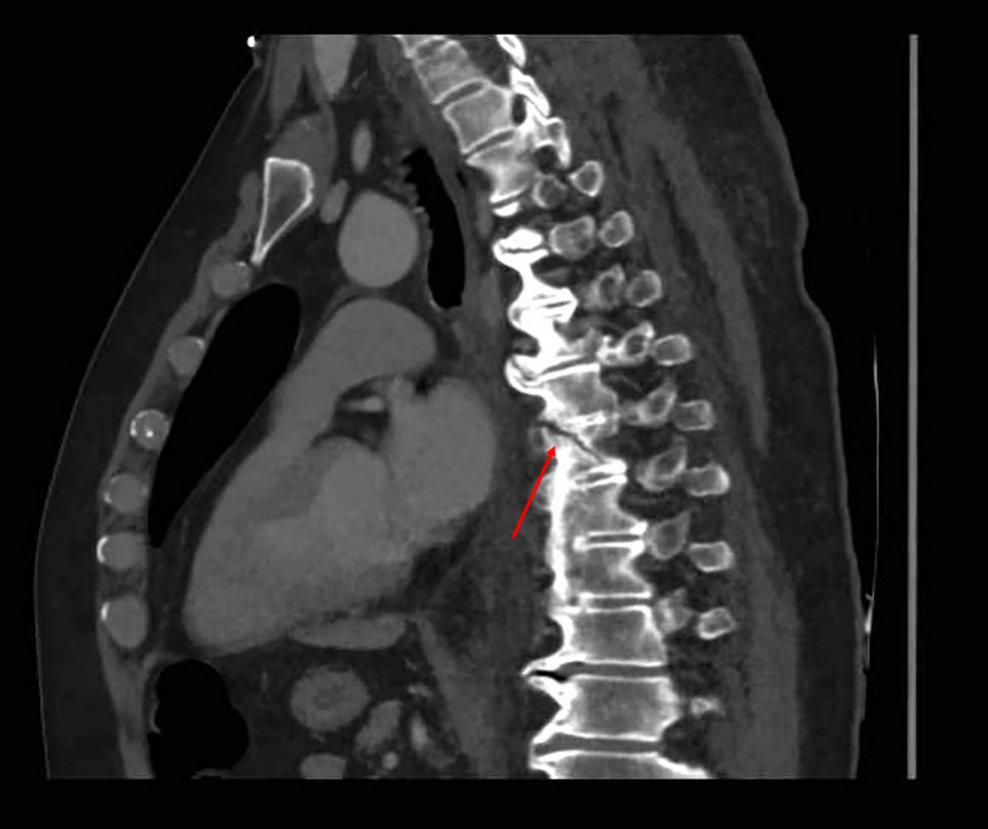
Chest computed tomography‐scan reveals an anterior vertebral fracture at level D8.
